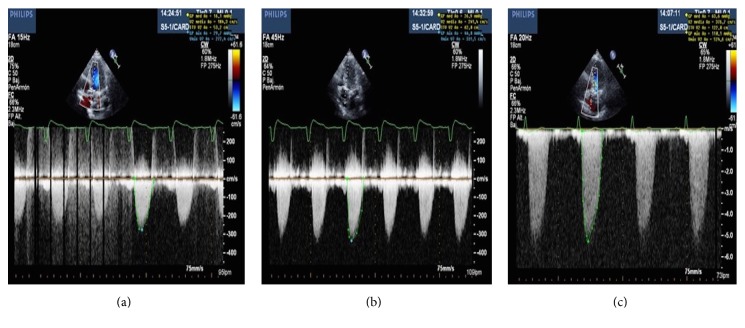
Patient with true low gradient severe aortic stenosis showing increased aortic valve gradient with continuous Doppler during dobutamine infusion at 10 g/kg/min (a), 15 g/kg/min (b), and 20 g/Kg/min (c). Aortic valve area remained below 0.5 cm^2^ during infusion.
